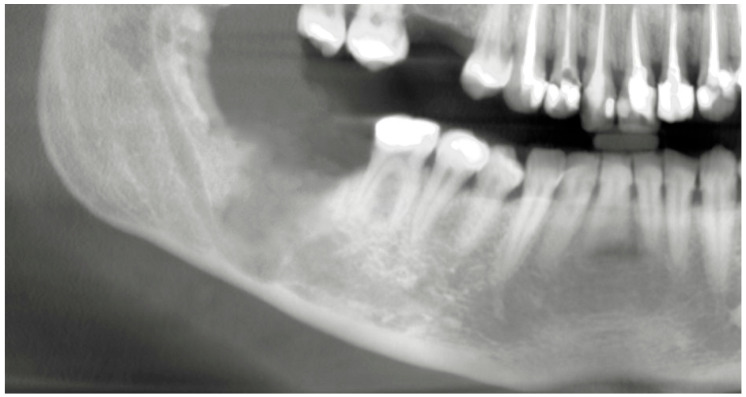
Six-month postoperative panoramic radiograph showing normal bone healing without radiographic evidence of lesion progression.
